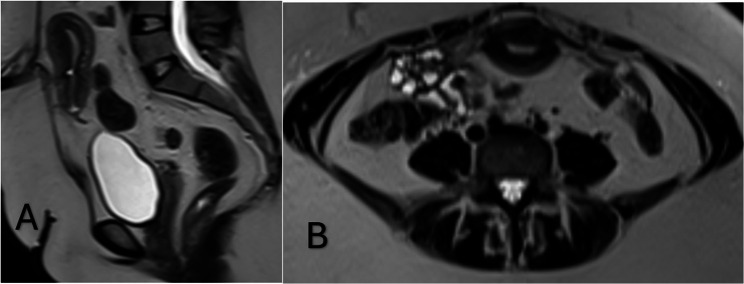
Sagittal (A) and Axial (B) T2W MRI showing the transpositioned uterus beneath the anterior abdominal wall at the level of the umbilicus with preserved zonal anatomy. Smooth vaginal stump. The transposed right ovary is also noted with follicular activity
